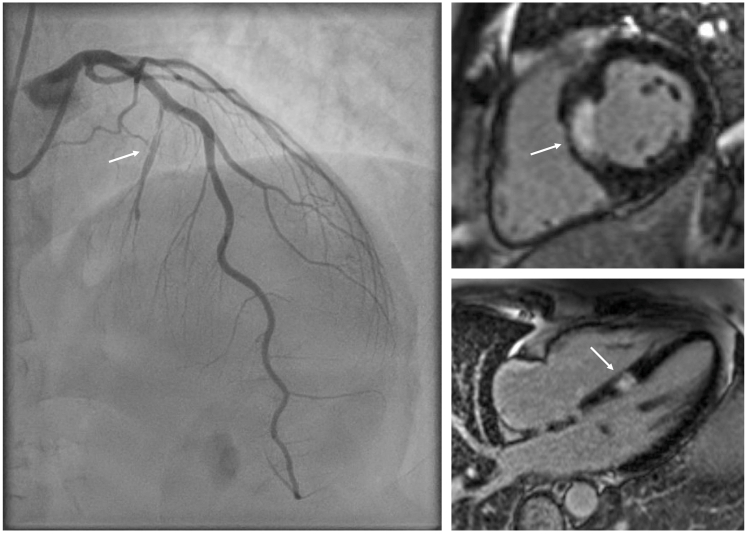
Isolated First Septal Perforator Spontaneous Coronary Artery Dissection Causing Acute Myocardial Infarction Type 1 spontaneous coronary artery dissection of the first septal perforator resulting in transmural infarction of the interventricular septum (arrows indicate the culprit vessel on angiography and corresponding infarct on cardiac magnetic resonance imaging).
